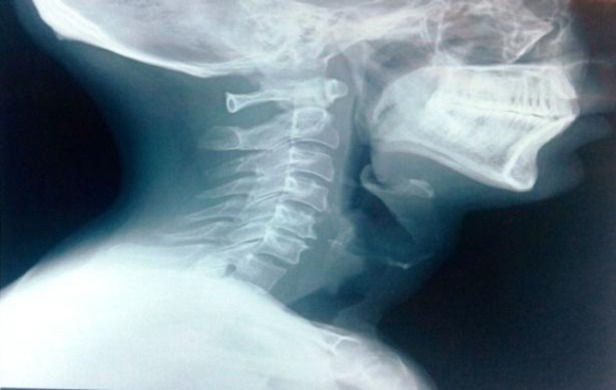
Lateral soft tissue neck radiograph demon- strating tracheal shadow containing vague opacity below the larynx.
